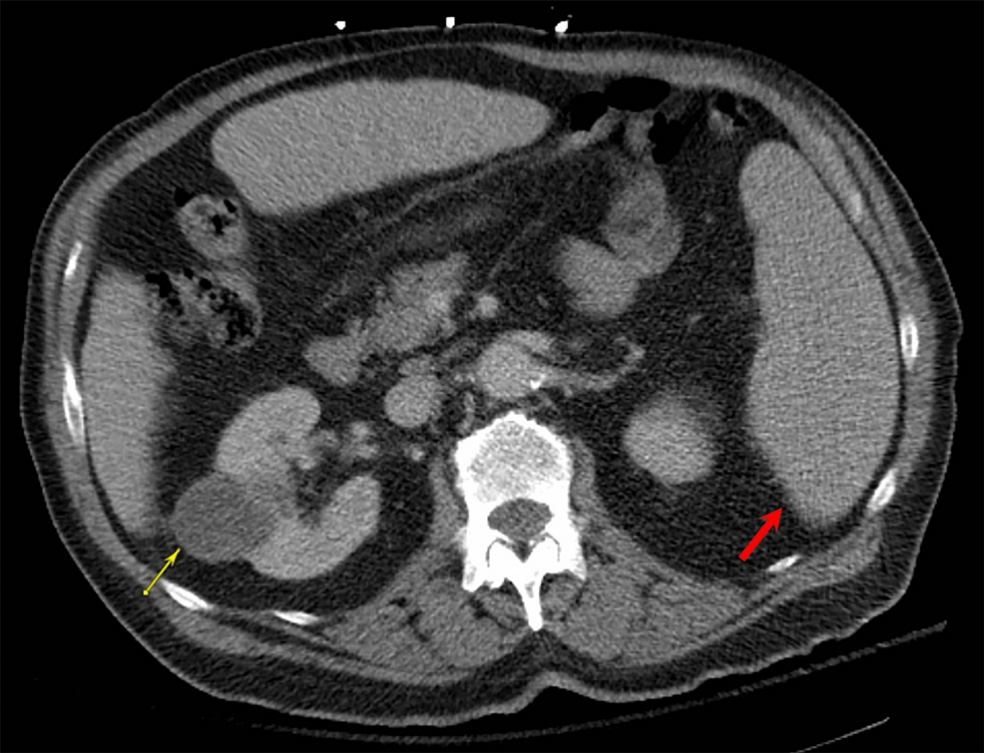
CT abomen and pelvis with IV contrast demonstrating splenomegaly (red arrow) and a right interpolar renal lesion (yellow arrow) measuring 2.7 cm consistent with renal cell carcinoma.
